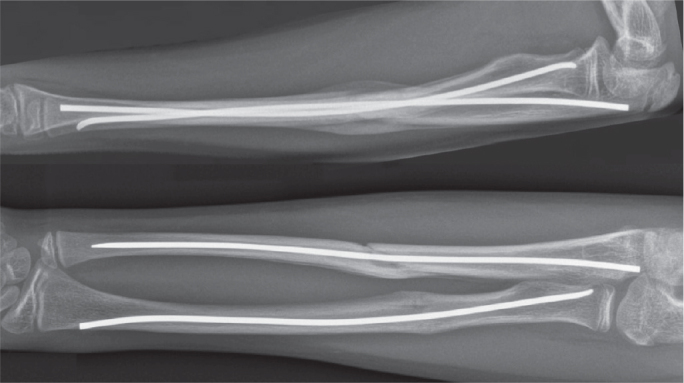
Postoperative lateral and anteroposterior radiographs of the forearm 6 weeks after elastic stable intramedullary nailing, showing fracture alignment with callus formation and adequate axial nail impaction in both the radius and ulna, with no extraosseous nail protrusion.
